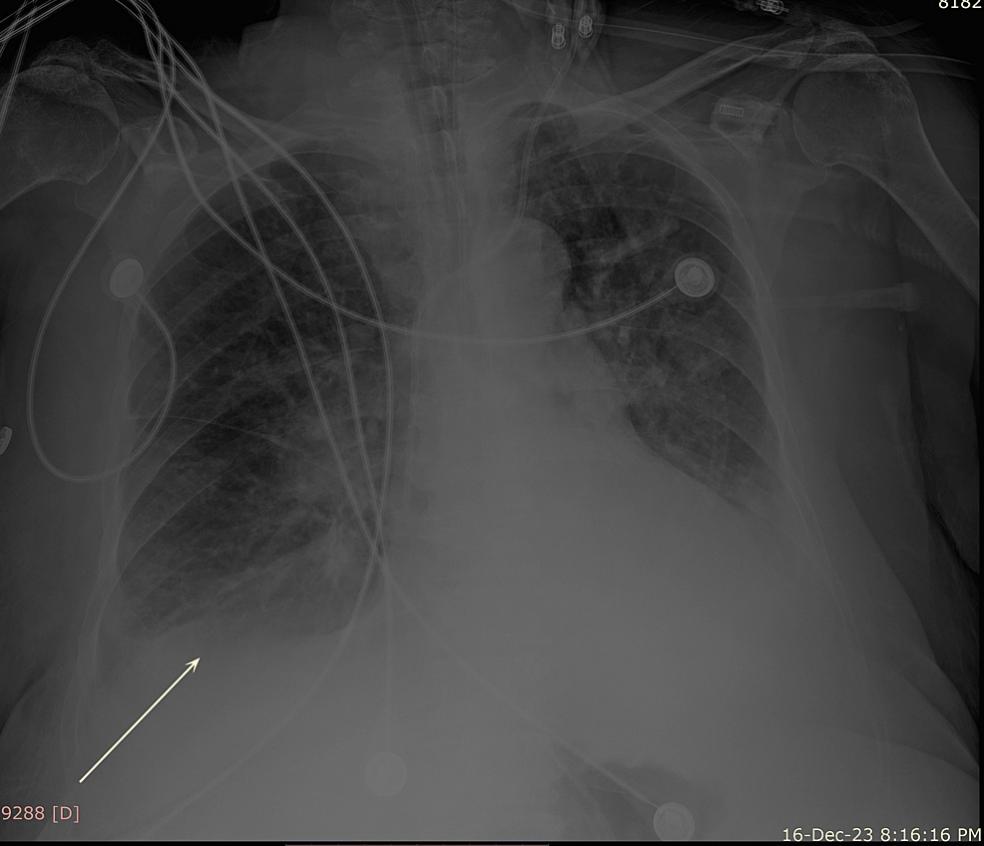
Postoperative portable chest X-ray showing resolution of the right lower lobe collapse and re-expansion of the lung (white arrow).
